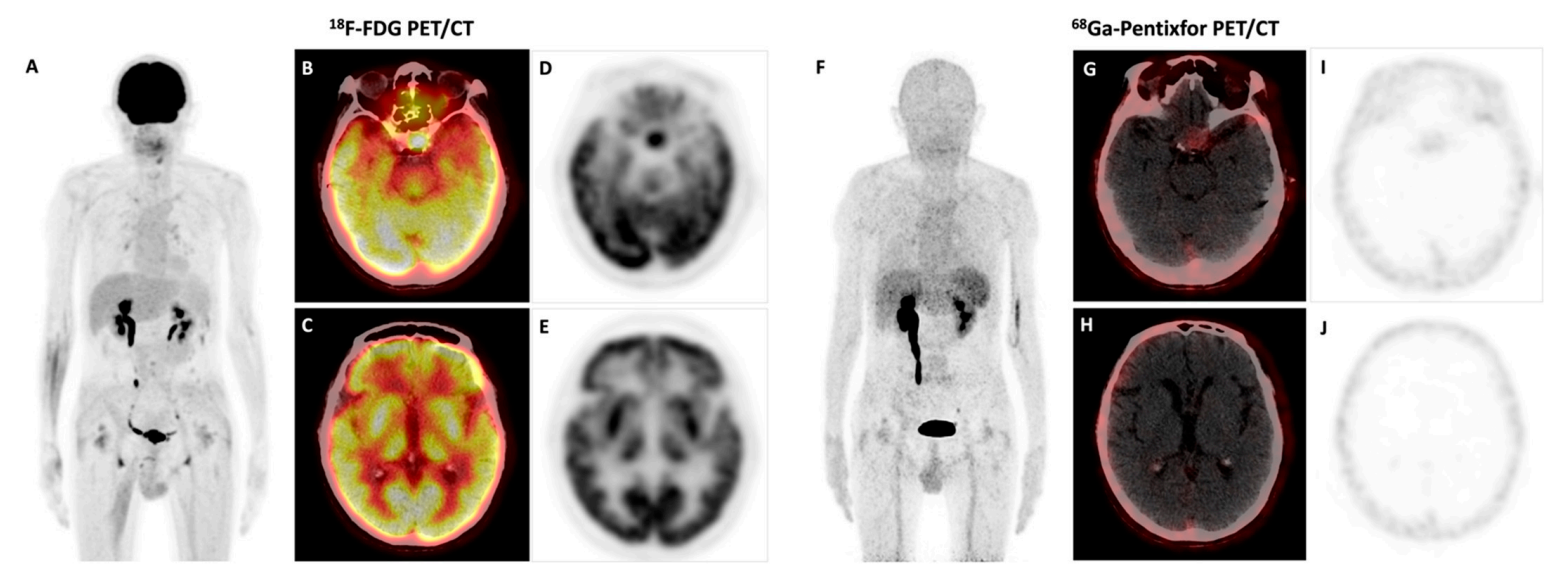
After six cycles of chemotherapy, the patient underwent follow-up PET/CT with both ^18^F-FDG and ^68^Ga-Pentixafor. MIP image of the ^18^F-FDG PET (A), axial fusion ^18^F-FDG PET/CT (B,C), axial ^18^F-FDG PET (D,E), MIP image of the ^68^Ga-Pentixafor PET (F), axial fusion ^68^Ga-Pentixafor PET/CT (G,H), and axial ^68^Ga-Pentixafor PET (I,J). The previous high uptake of ^68^Ga-Pentixafor in the choroid plexus returned to normal. There was also a complete remission of bone marrow, lymph node, and nerve roots involvement in both ^68^Ga-Pentixafor and ^18^F-FDG PET/CT. However, the manifestations of the pituitary macroadenoma remained unchanged (SUVmax 11.2 in ^18^F-FDG PET/CT). Bing–Neel syndrome is a rare complication involving almost 1% of WM patients [4]. About one-third of patients with Bing–Neel syndrome had symptoms of peripheral neuropathies [4,5]. Leptomeningeal infiltration was most commonly seen, followed by parenchymal and dural involvement [4,6]. Involvement in the choroid plexus is rare. MRI can be used to detect central nervous system involvement in Bing–Neel syndrome, but it lacks specificity. In a previous case report of Bing–Neel syndrome, the lesions shown in MRI were hypometabolic in ^18^F-FDG PET/CT [7], which was consistent with our case. Two other cases reported FDG-avid central nervous system involvement of Bing–Neel syndrome [8,9]. ^18^F-FDG showed pronounced physiological accumulations in the brain, which may omit the pathological findings. However, the physiologic uptake of ^68^Ga-Pentixafor is very low in the head, resulting in excellent tumor-to-background contrast and a higher detection rate of cerebral lesions. Thus, as there is a high level of CXCR4 expression in B-cells of WM [1,10], ^68^Ga-Pentixafor should be further assessed as a potential tracer for the evaluation of WM, including the involvement of the central nervous system.
